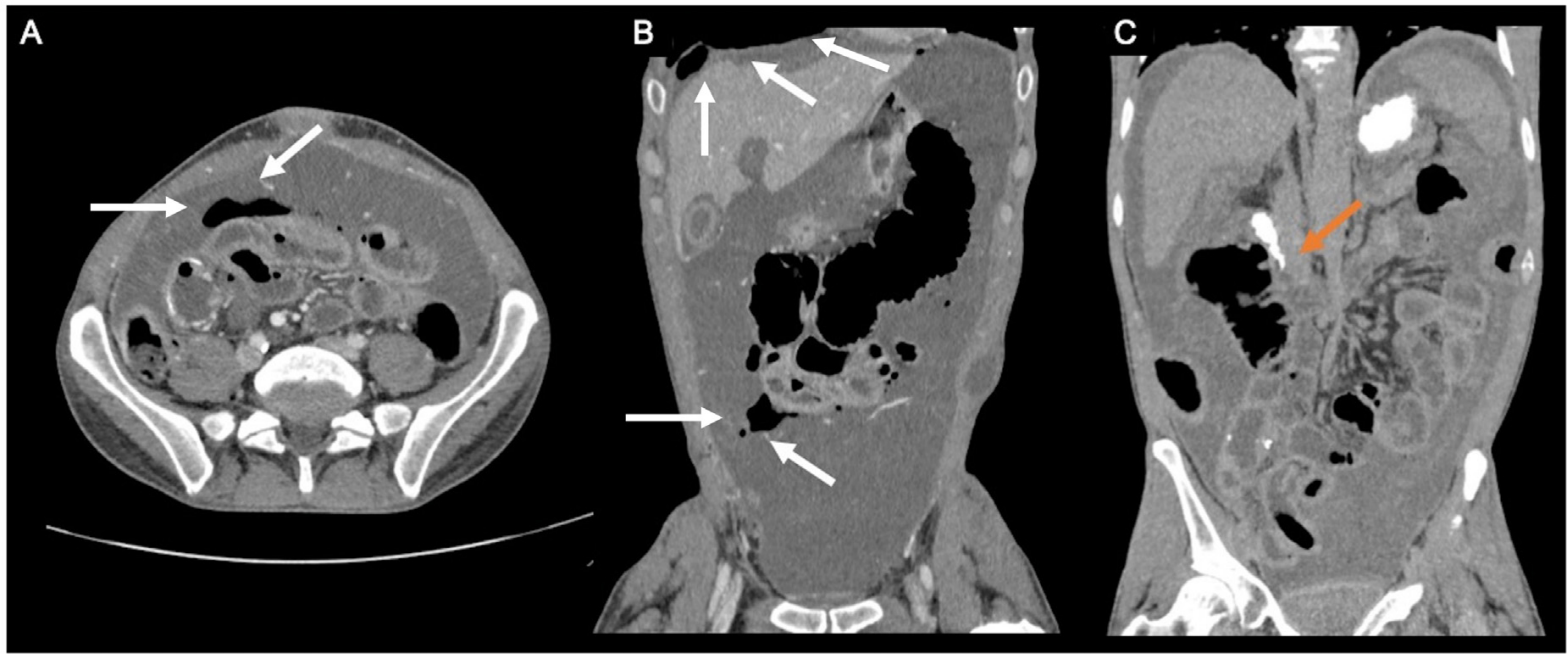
Contrast enhanced CT scan of the abdomen and pelvis, on axial (A) and coronal (B-C) planes, the latter (C) after oral administration of iodinated oral contrast. The pictures show massive neoplastic ascites and pneumoperitoneum (white arrows). On C no progression of the iodinated oral contrast is seen beyond the second portion of the duodenum (arrow).
